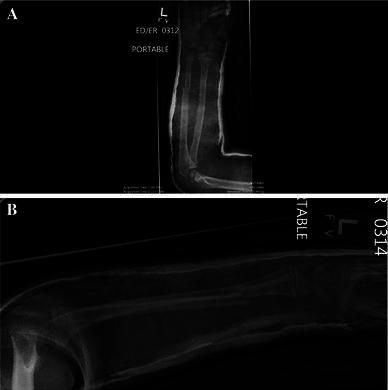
a, b Immediate post-reduction radiographs in the emergency department demonstrating satisfactory reduction of the fractures in a long arm cast
